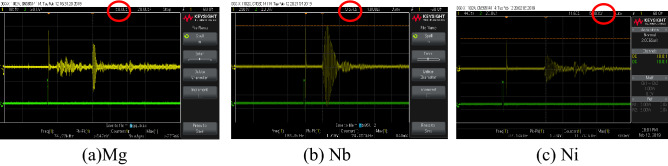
The ultrasonic test for Mg, Nb, and Ni.
